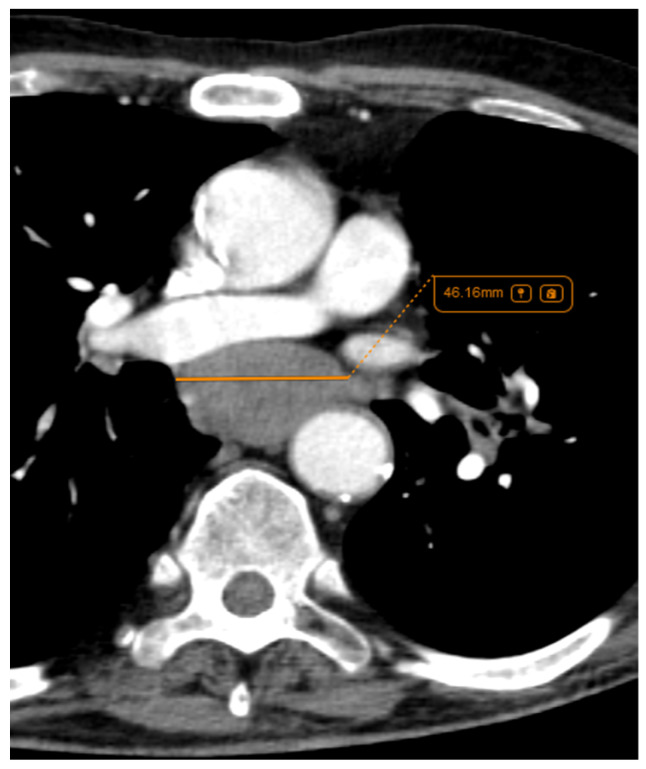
Axial CT—soft tissue view of a lymph node localised in the infracarinal region, from a left peripheral ADC.
